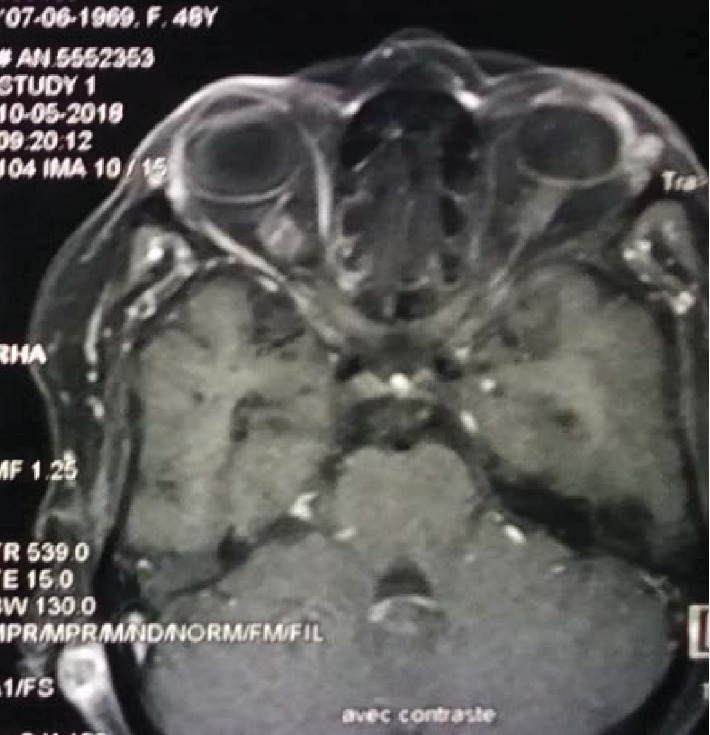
MRI objectifying a voluminous cerebellar lesion enhanced after gadolinium injection with temporal left scleral thickening.
